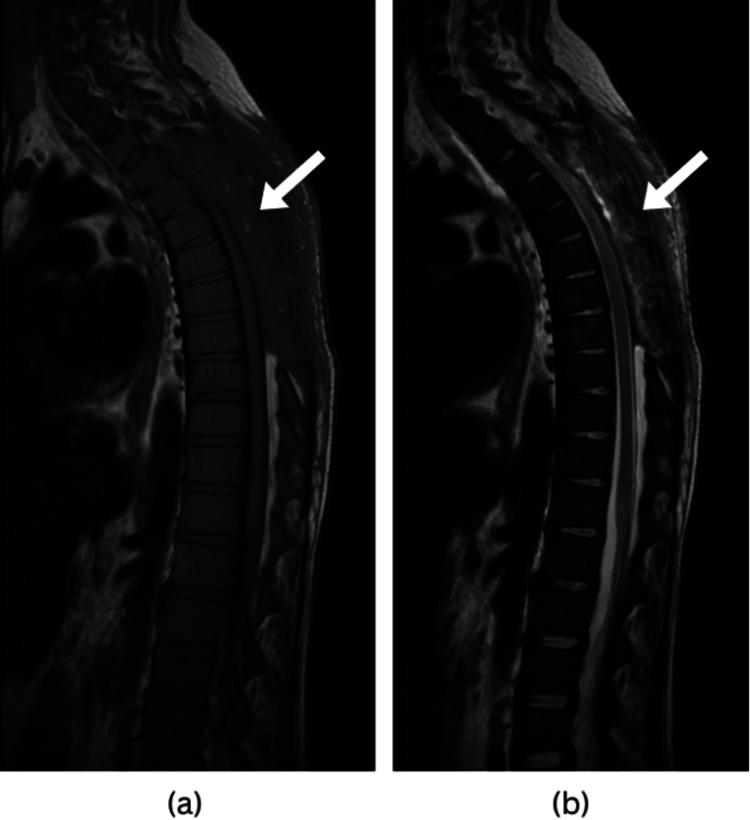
Post-op MRI of the dorsal spine. Sagittal T1-weighted image (a) and sagittal T2-weighted image (b), a laminectomy is evident, with the spinal processes absent from T3 to T8 (indicated by arrows), along with the removal of the previously observed epidural lesion. There is no evidence of spinal cord compression.
